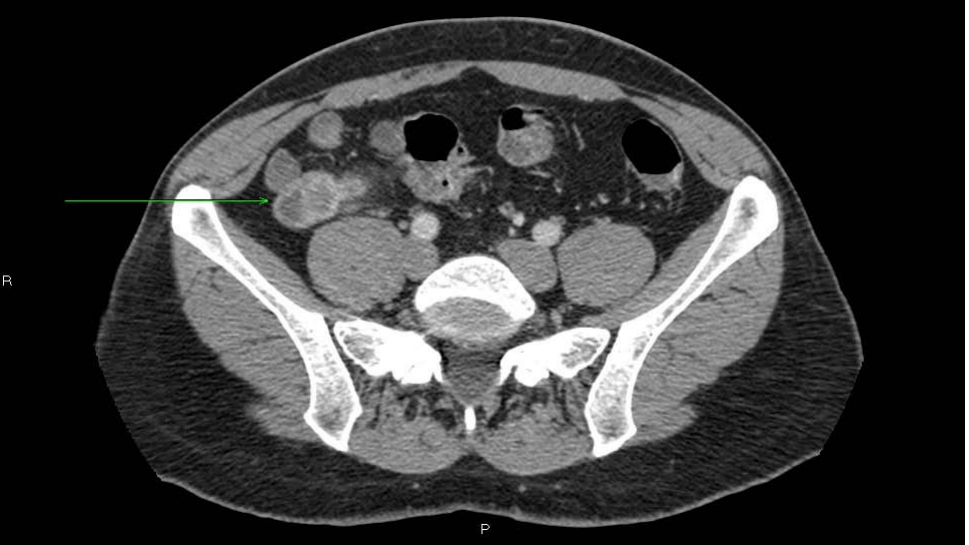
CT enterography. Green arrow shows a mass lesion arising from either the appendix or a Meckel's diverticulum.
